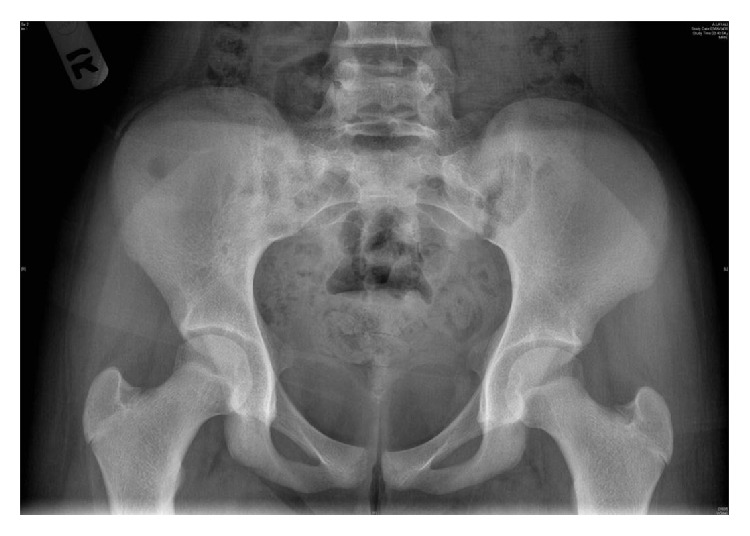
X-ray AP view of the pelvis showing hard to pick changes in the right ilium.
